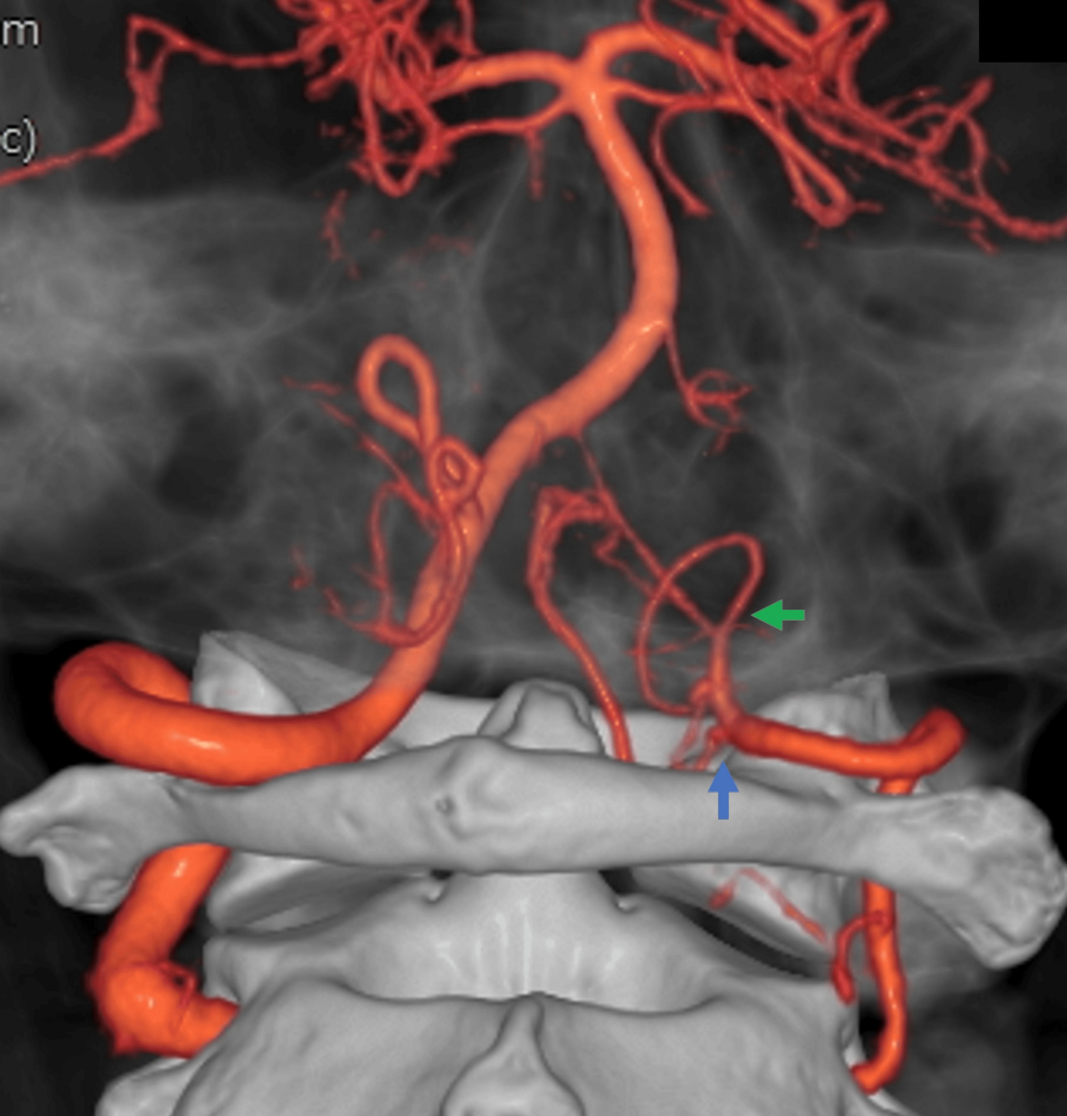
3D CT angiography revealed the proximal origin (blue arrow) arising from the distal part of the V3 segment and the distal origin (green arrow) emerging from the proximal part of the V4 segment
